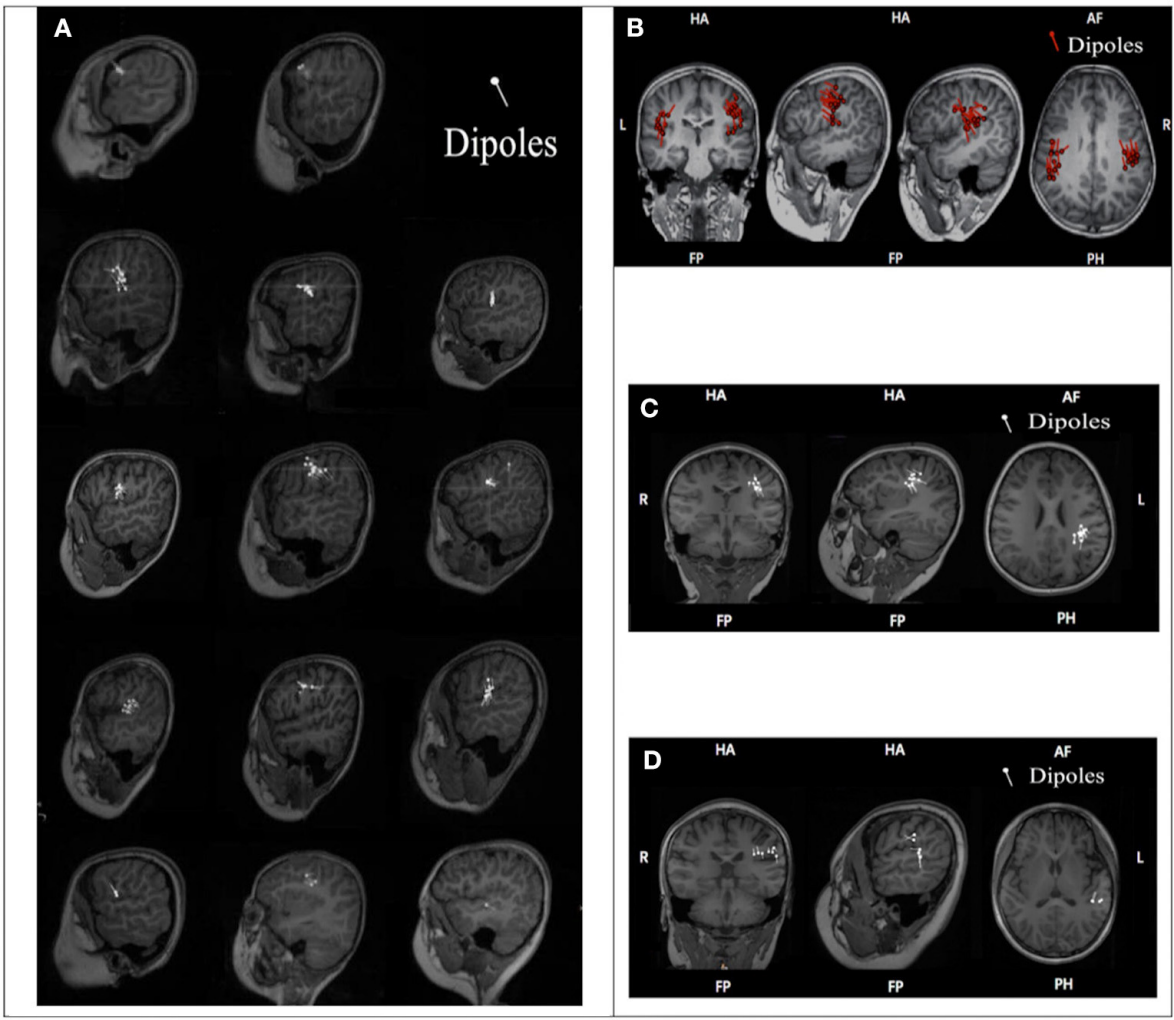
ELP4 rs662702 genotypes and CTS source distributions in 17 patients. (A) Genotype and distribution of CTS sources (dipoles) in 14 BECTS patients. Line 1: Two BECTS patients were ELP4rs662702 CC homozygous and their CTS sources were located in the frontal lobe. Lines 2–4: Nine BECTS patients were ELP4rs662702 CC homozygous and their CTS sources were mainly located in the central region. Line 5: Three BECTS patients were ELP4rs662702 CT homozygous and their CTS sources were located in the frontal gyrus, middle frontal gyrus, and superior temporal gyrus (from left to right). (B) The CSWS patient was ELP4rs662702 CC homozygous and his CTS sources were located in the bilateral inferior pre-central gyrus. (C) The ABPE patient was ELP4rs662702 CC homozygous and his CTS sources were located in the left inferior pre-central gyrus. (D) The ABPE patient was ELP4rs662702 CC homozygous and his CTS sources were located in the inferior post-central gyrus and superior temporal gyrus.
